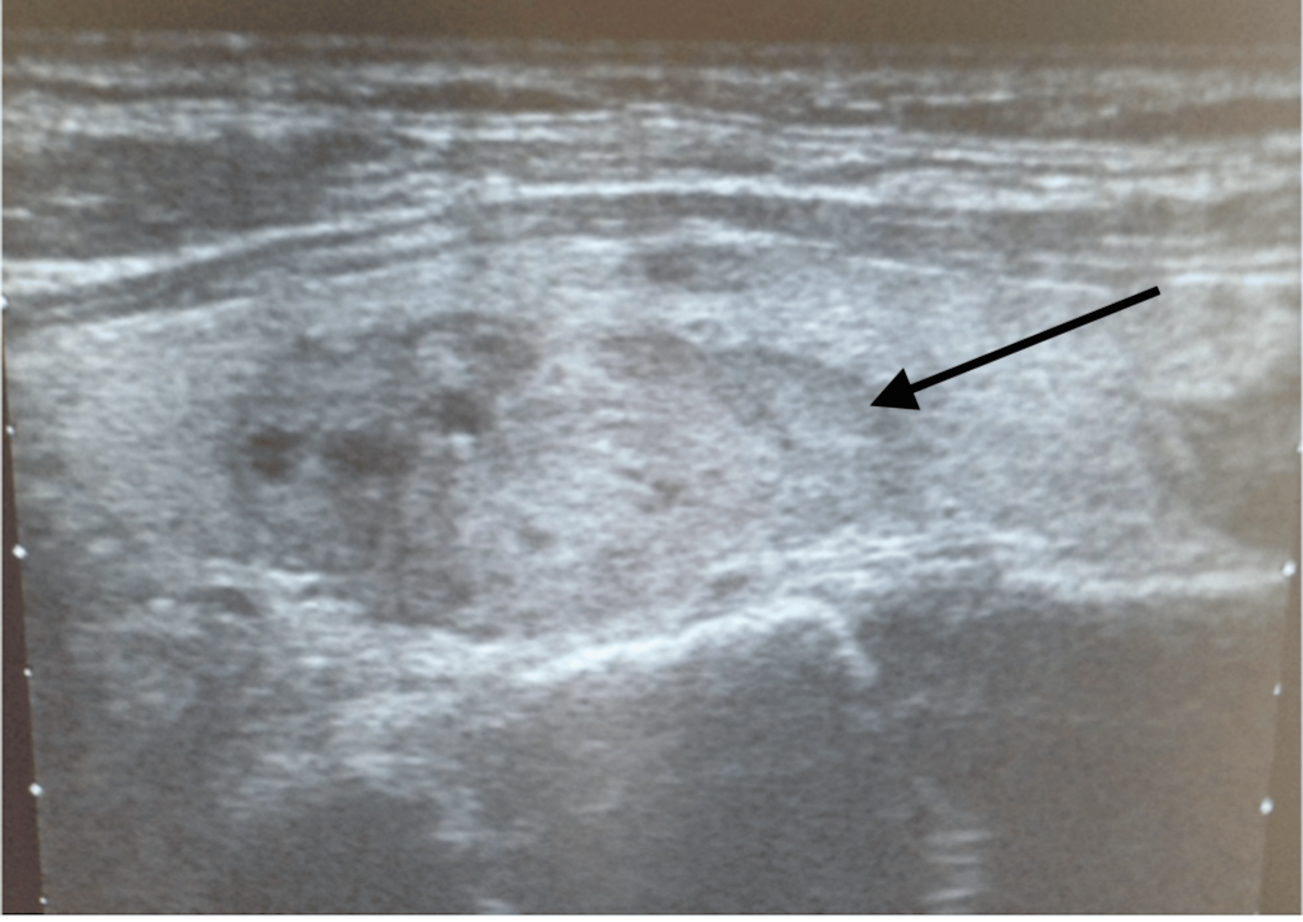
Neck US, 16x10cm hypoechoic nodule in left inferior thyroid lobe. Black arrow shows a hypoechoic nodule surrounded by thyroid gland. US: Ultrasound
